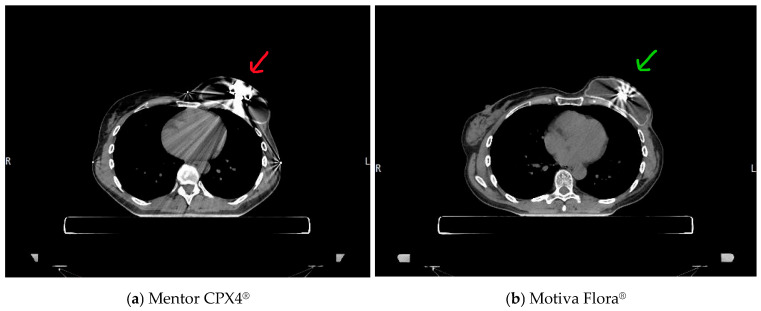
Representative axial CT images showing artifact generation from tissue expanders: (a) Mentor CPX4^®^ expander with metallic port, demonstrating pronounced beam-hardening artifacts; (b) Motiva Flora^®^ expander with non-metallic RFID port, showing reduced artifact burden.
